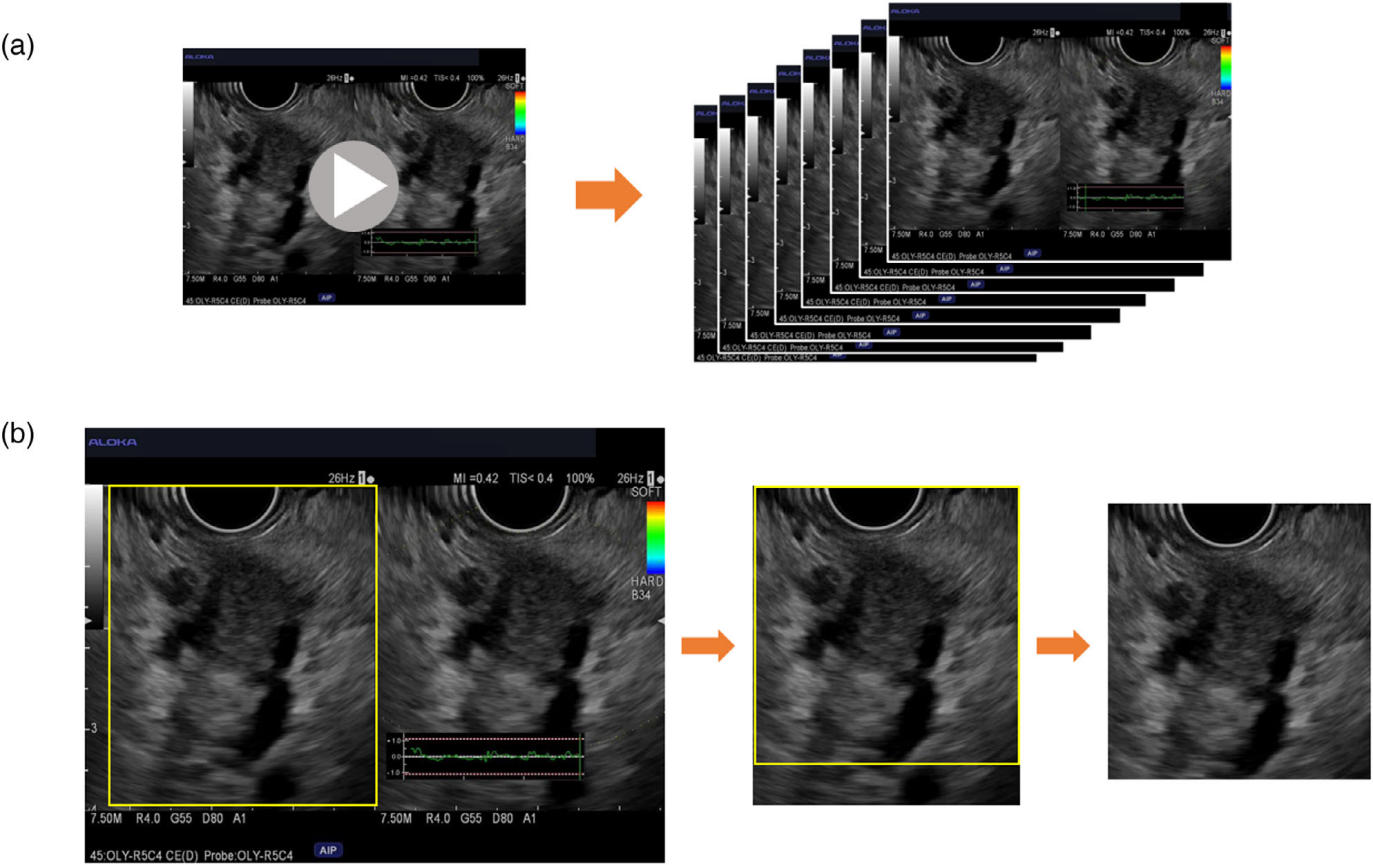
Endoscopy ultrasonography image preparation. (a) Prior to endoscopic ultrasound‐guided fine needle aspiration/biopsy of the pancreas, the lesions were observed and recorded for approximately 10 s. From these videos, all images of the pancreatic lesion were stored as digital still images (JPEG format) for each patient. (b) All endoscopic ultrasound images were uniformly cropped to remove potentially confusing image features and identifying information. This way, unbiased and anonymous images measuring 465 × 489 pixels constituted our dataset.
